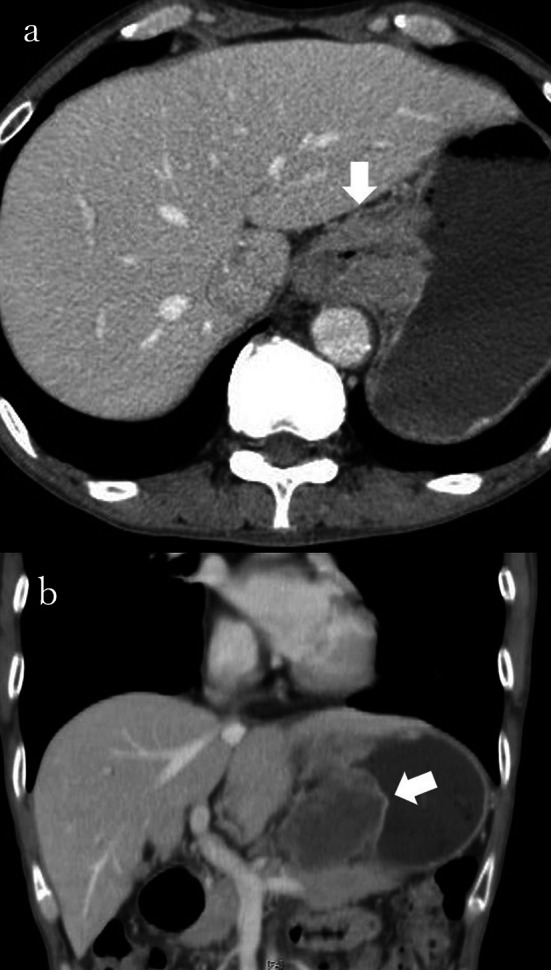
Findings of computed tomography. a Thickened wall as primary lesion was observed from the lower esophagus to fundus of stomach (arrow). b A solitary metastatic abdominal lymph node measuring 56 mm made a mass and invaded to lesser curvature and pancreatic body (arrow)
